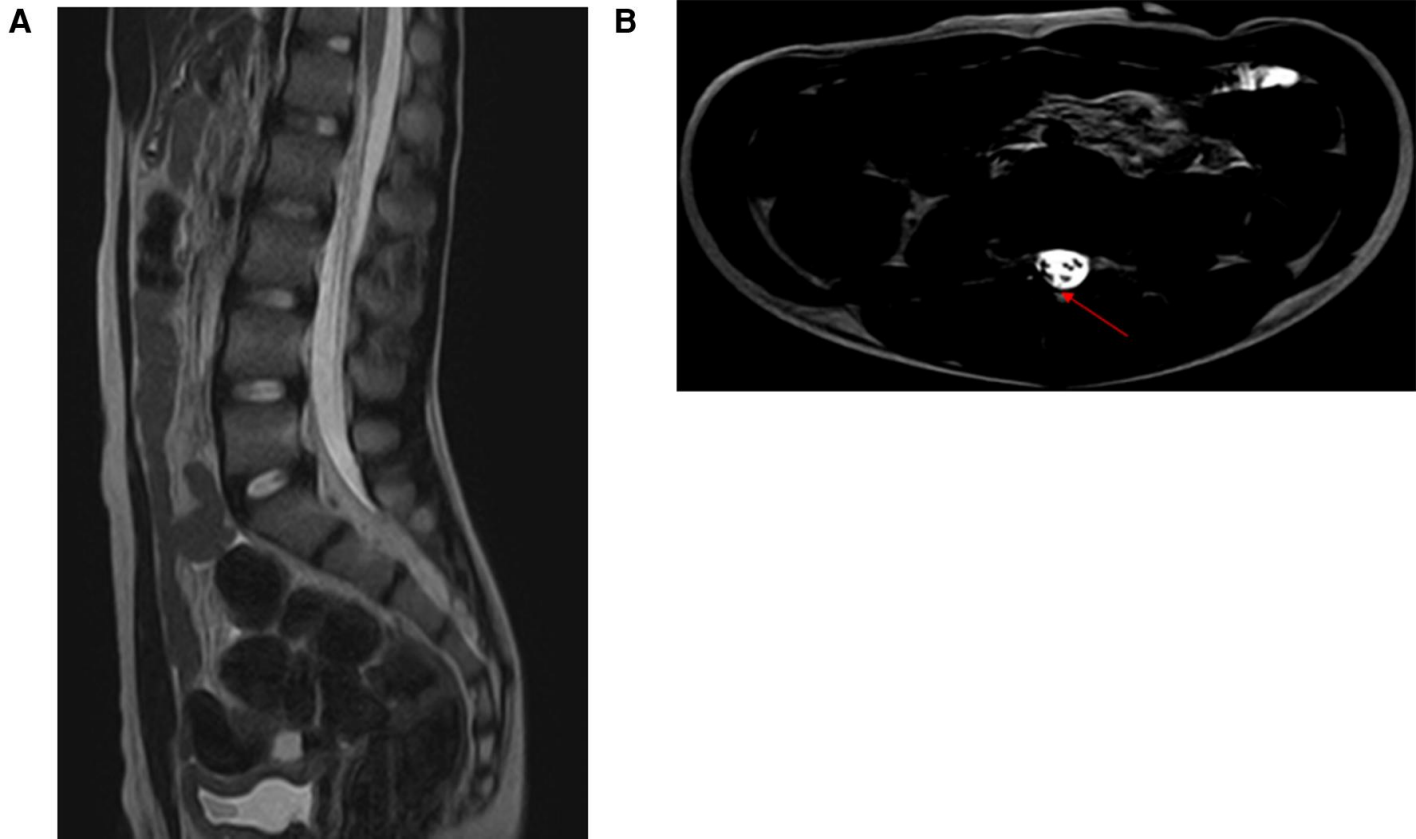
(A,B) MRI of the lumbosacral spine in the prone position revealed that the filum terminale was thickened and deviated dorsally, suggesting the possibility of tight filum terminale.
